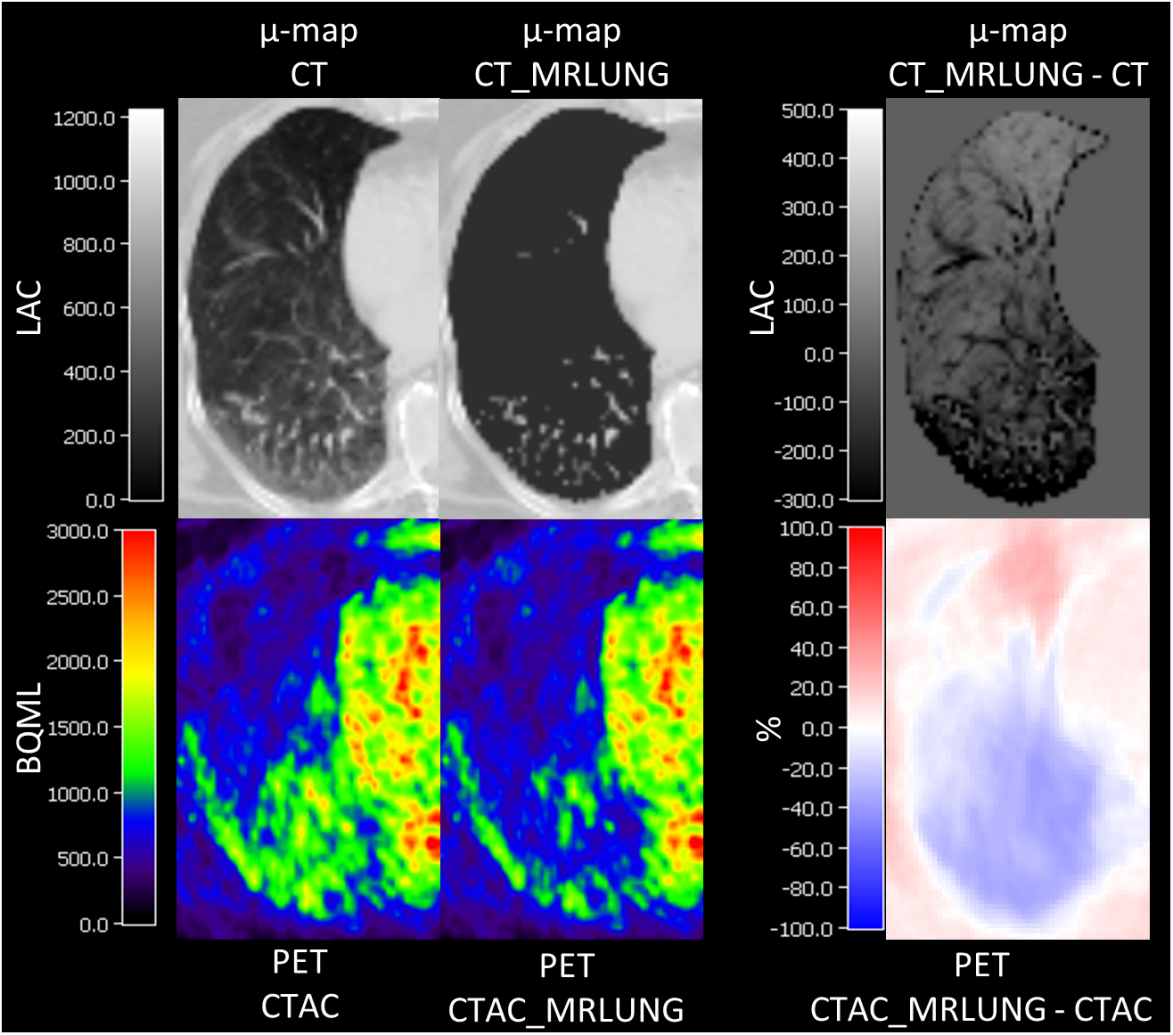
Typical example of an axial view of the right lung of a patient to demonstrate the effect of the replacement of the CT-based LACs of lung tissue (μ-map CT) with the LAC from the MR-based segmentation method from the Biograph mMR (μ-map CT_MRLUNG). To show the differences of LACs, a subtraction map is given in the upper row on the right-hand side (μ-map CT_MRLUNG—CT); as one can see, the subtraction of μ-map CT from μ-map CT_MRLUNG leads to an underestimation of LACs in the posterior regions and an overestimation in the anterior regions (given in LACs). This results in higher SUVs in PET_CTAC as compared to PET_CTAC_MRLUNG in the posterior regions, or, in other words, an underestimation of SUVs in the posterior regions of PET_CTAC_MRLUNG as shown in the subtraction image PET CTAC_MRLUNG–CTAC.
